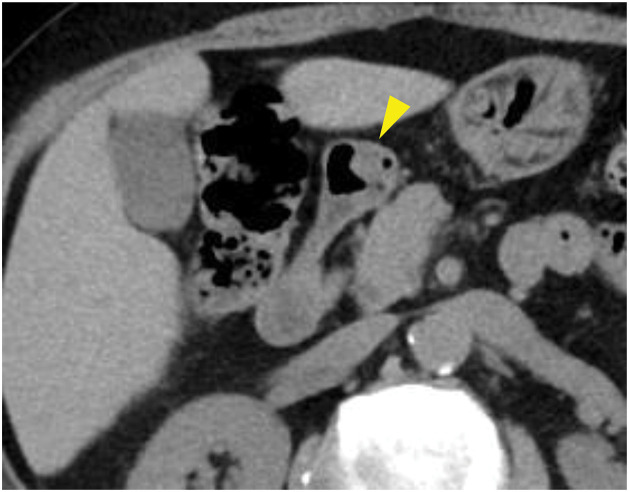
Findings of computed tomography. Computed tomography scan image. No lesions were detected in the duodenal bulb (yellow arrowhead).
